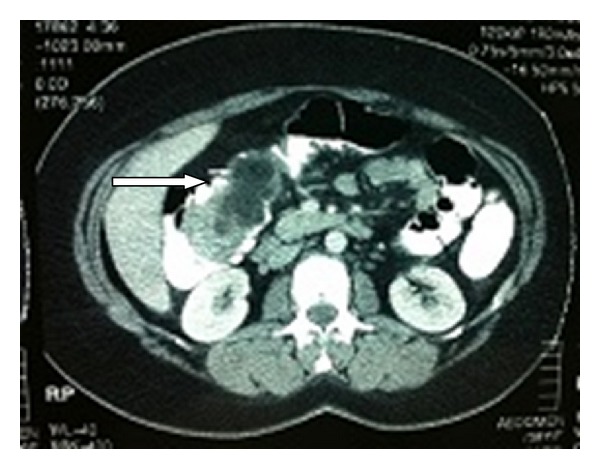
Contrast-enhanced abdominal CT revealing the presence of an ovoid endoluminal mass extending from the cecum to ascending colon with fatty density (arrow) without extramural extension.
